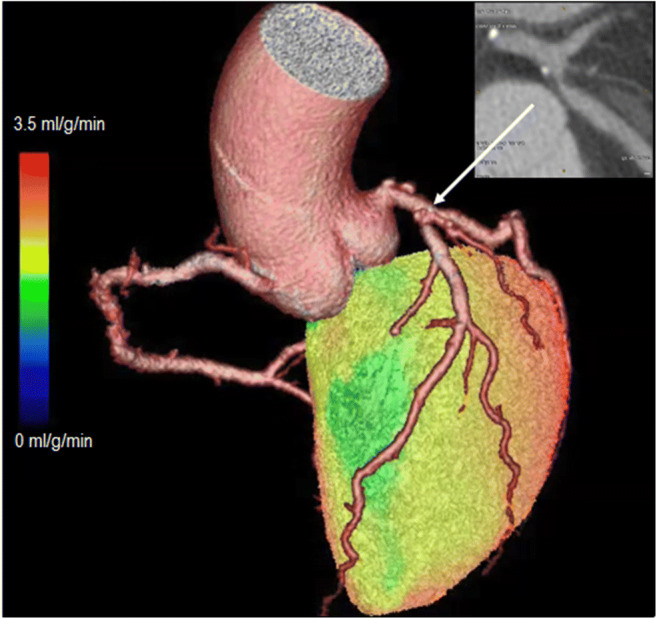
Hybrid PET/CT image demonstrating 3D reconstruction of coronary anatomy and MBF. CCTA shows a stenosis in the proximal left anterior descending coronary artery (insert), and [^15^O]water PET shows reduced MBF (green colour) in the myocardium subtended by the artery during adenosine stress
